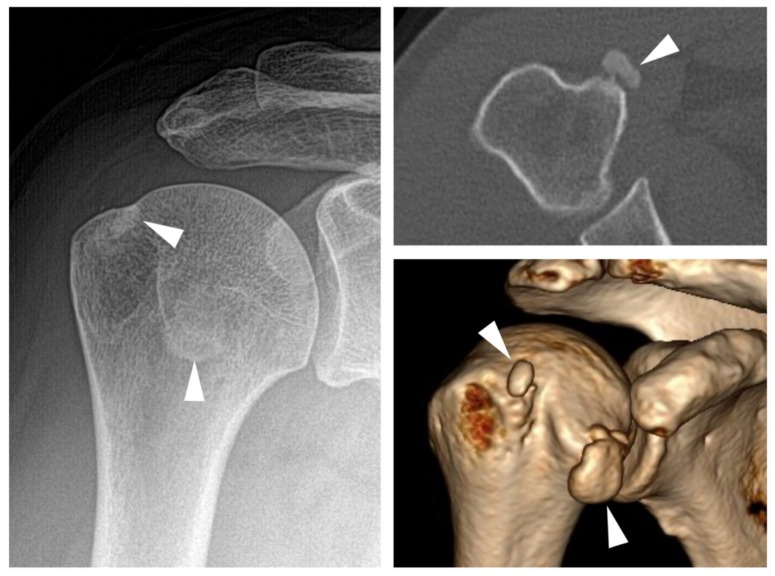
BCP deposition. (Left): White arrowheads indicate calcific deposition on radiography. (Right): Axial and 3D reconstructions of the same shoulder with better visualization of the depositions.
